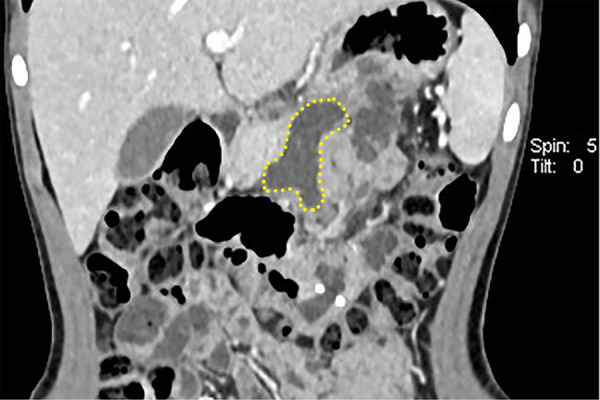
(Case 3): follow-up CT scan 11 days postoperatively shows localized fluid collection around the anastomosis (yellow circle).
